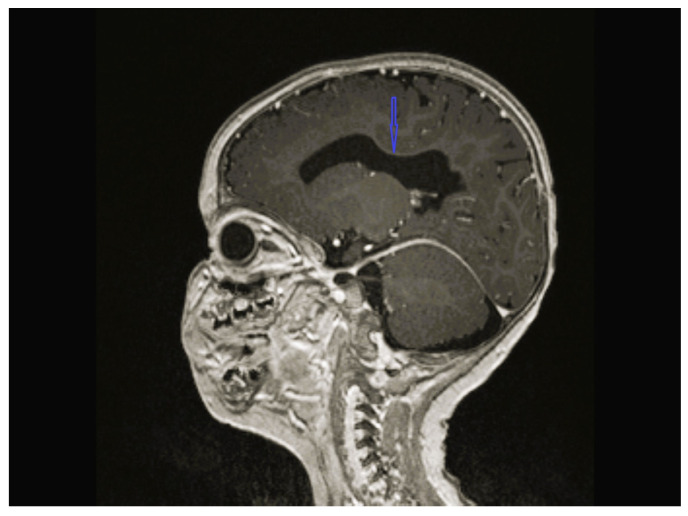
Brain MRI of case 2 revealing hypoplasia of the corpus callosum.
